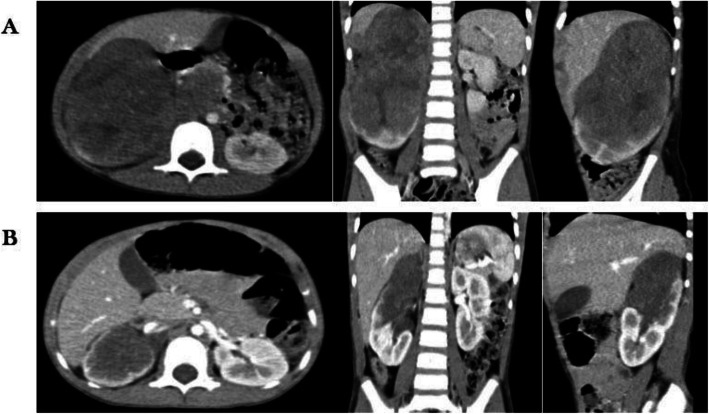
CT abdomen showing right Wilms’ tumor locally advanced. a Initial imaging at presentation. b Imaging post 6 weeks of chemotherapy showing regressive course
